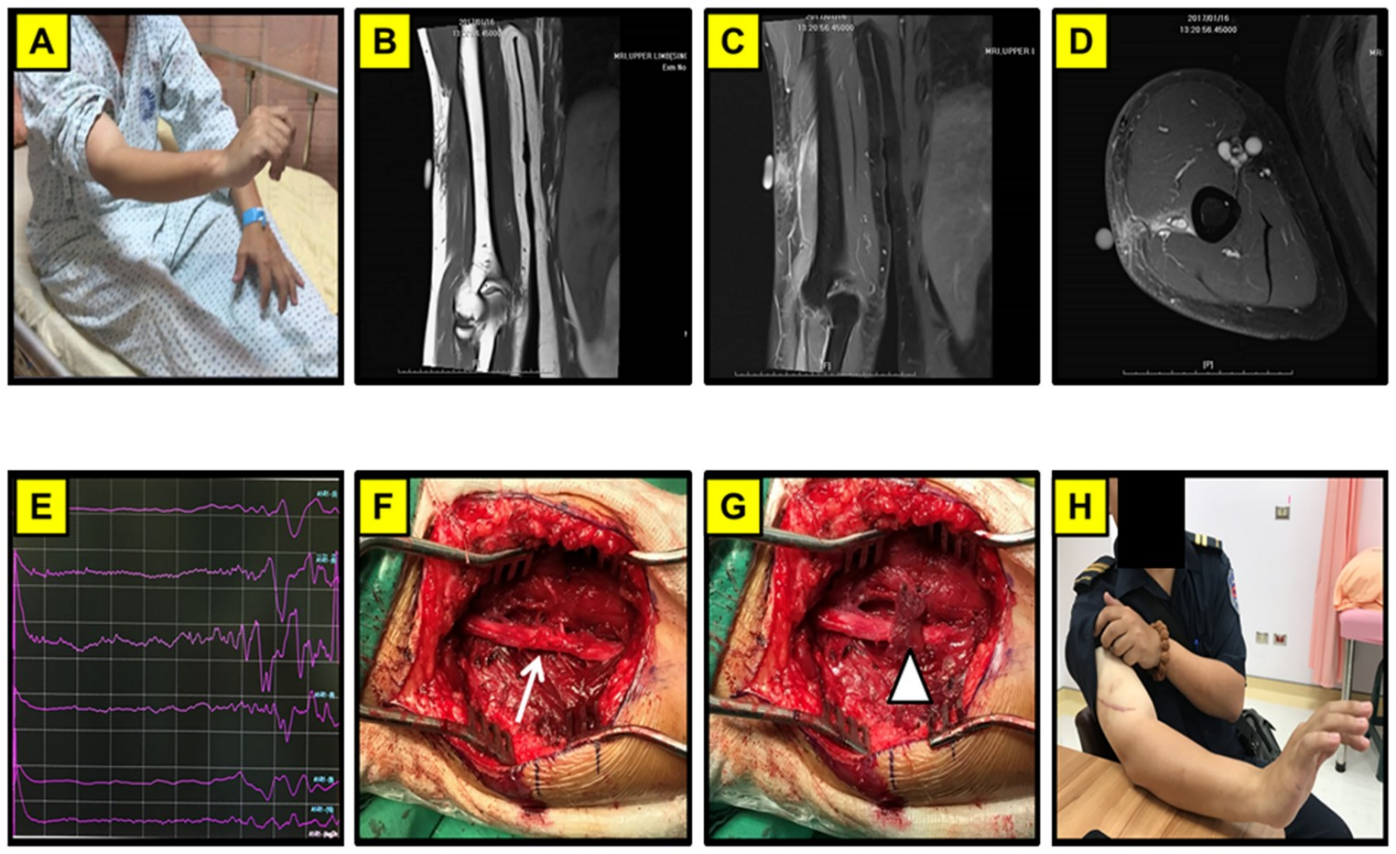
Representative right radial nerve injury by a crush injury with chronic denervation. (A) A 38-year-old male suffered a crush injury with the paralysis of finger dorsal flexion for 6 months. (B) MRI of right upper limb in T1W1 in coronal axial view. (C) MRI of right upper limb in T1W1 with contrast administration in coronal view. (D) MRI of right upper limb in T1W1 with contrast administration in axial view. (E) Intraoperative recording of CMAP in brachioradials and dorsal digitorum of radialis. (F) Intraoperative photography showing nerve crushed injury. (G) The injured nerve wrapped by the biceps muscle. (H) The patient showed the improved neurological outcome with restoration of wrist function 2 months after the operation. Arrow: radial nerve in the crush site; Arrow head: muscle flap rotation.
